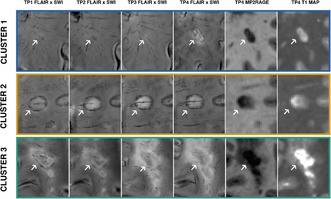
FIGURE 1 Longitudinal examples of metabolically clustered lesions (Clusters 1–3) across coregistered FLAIR + SWI, MP2RAGE, and quantitative T1 maps from baseline to 8‐year follow‐up.
